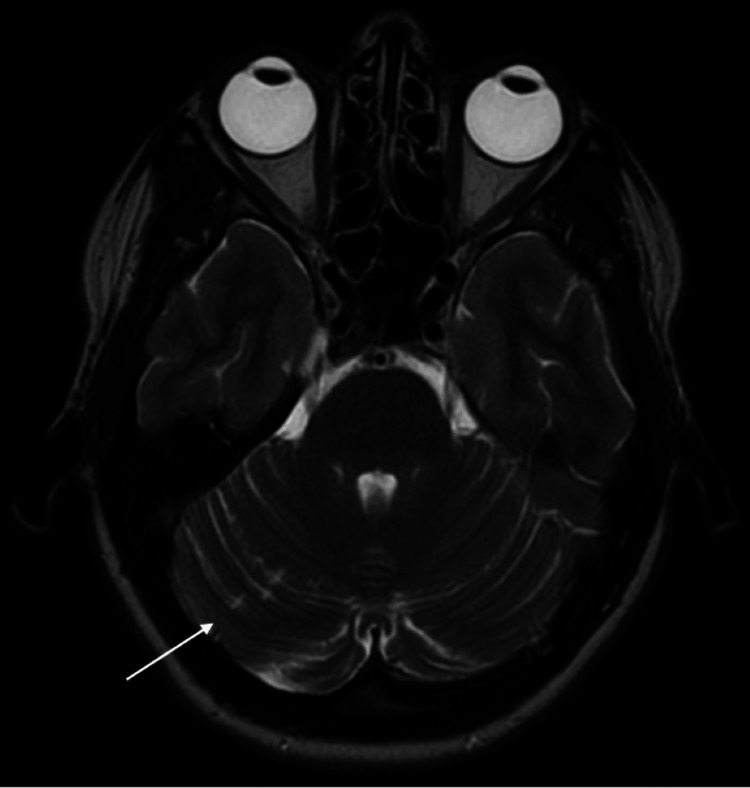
Follow-up MRI Head (axial view) demonstrating multiple small foci in right cerebellar hemisphere, no progression since previous MRI.
